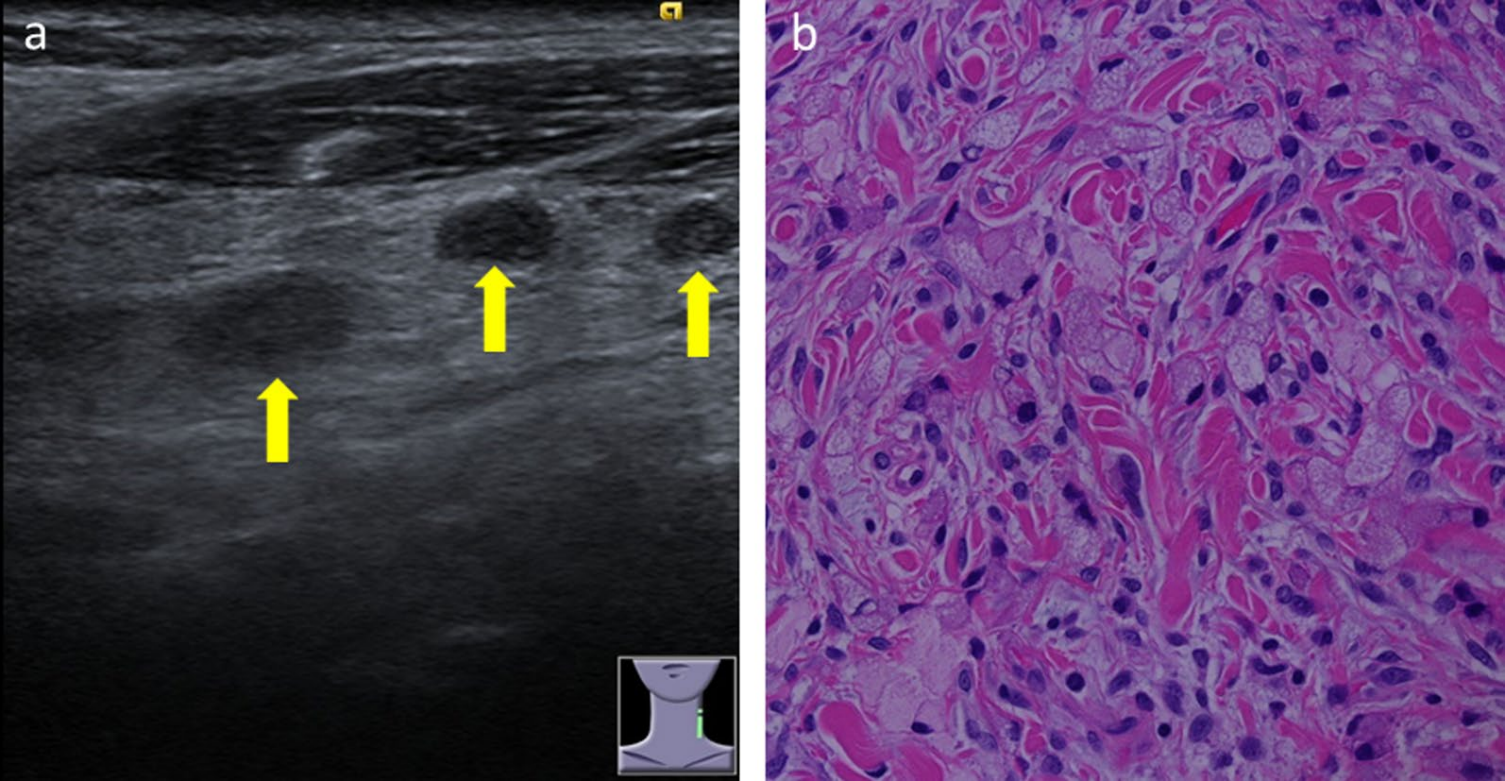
Ultrasonography and pathological findings in cervical lymph nodes. a Ultrasonography showed three pieces of lymph node enlargement in left cervical area (arrow). b Pathological findings revealed signet-ring cell and a poorly differentiated adenocarcinoma (× 400 magnification)
